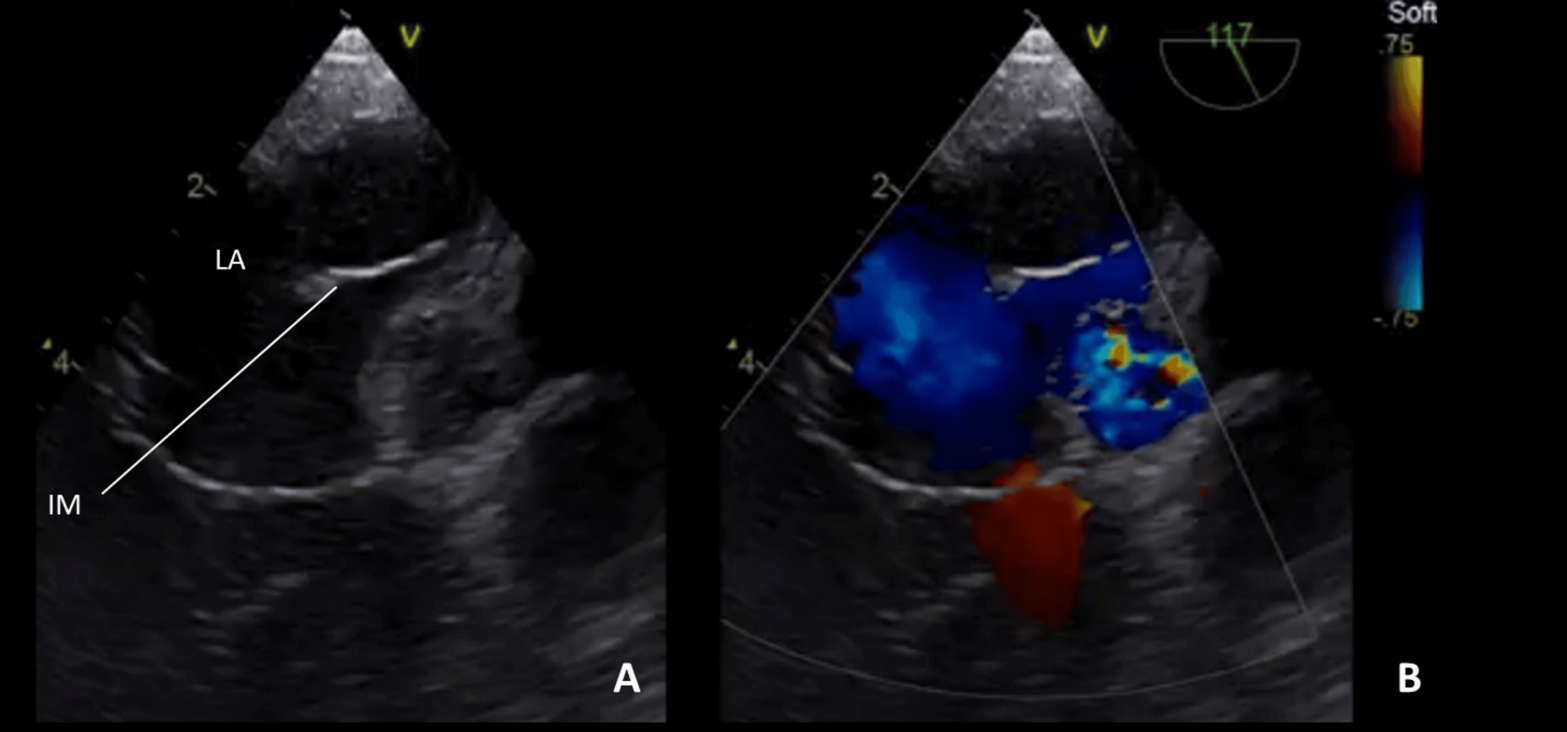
Cor triatriatum sinister, incomplete and non-obstructive. (A) Transesophageal echocardiography at 117° shows an incomplete membrane (IM) dividing the left atrium (LA) into two compartments. (B) Same view as in (A) with color Doppler demonstrating laminar flow between the compartments.
